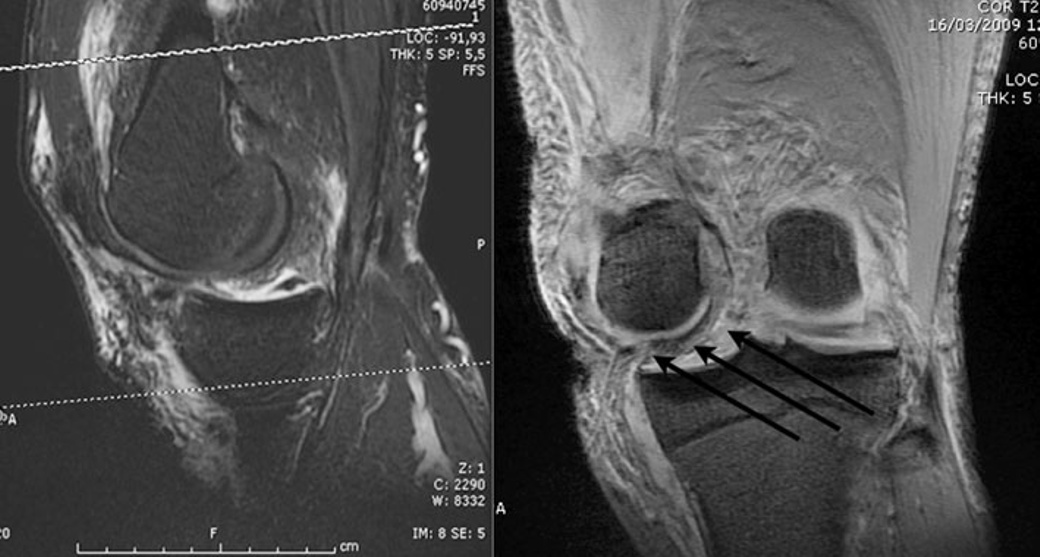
MRI showing muscular-density-like structure (indicated by*arrows*), which turned out to be intra-articularly dislocated
